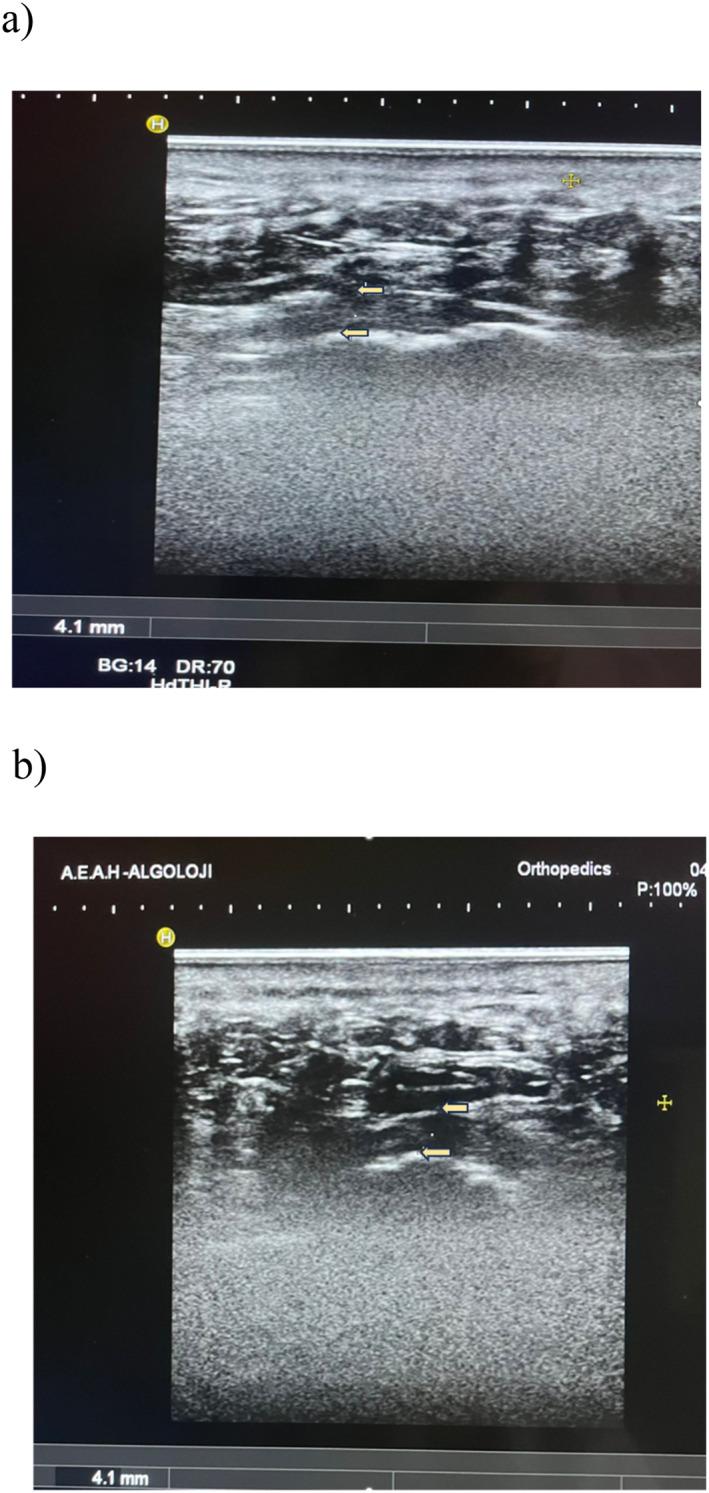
Ultrasonographic evaluation of plantar fascia thickness (PFT) in Patients with chronic plantar fasciopathy. (a) Longitudinal ultrasound image of the plantar fascia in a patient with recalcitrant plantar fasciitis, demonstrating thickening (4.1 mm) and hypoechoic changes near the calcaneal insertion. (b) Transverse ultrasound image of the plantar fascia in the same patient with recalcitrant plantar fasciitis, revealing persistent thickening (4.1 mm) at the calcaneal insertion.
